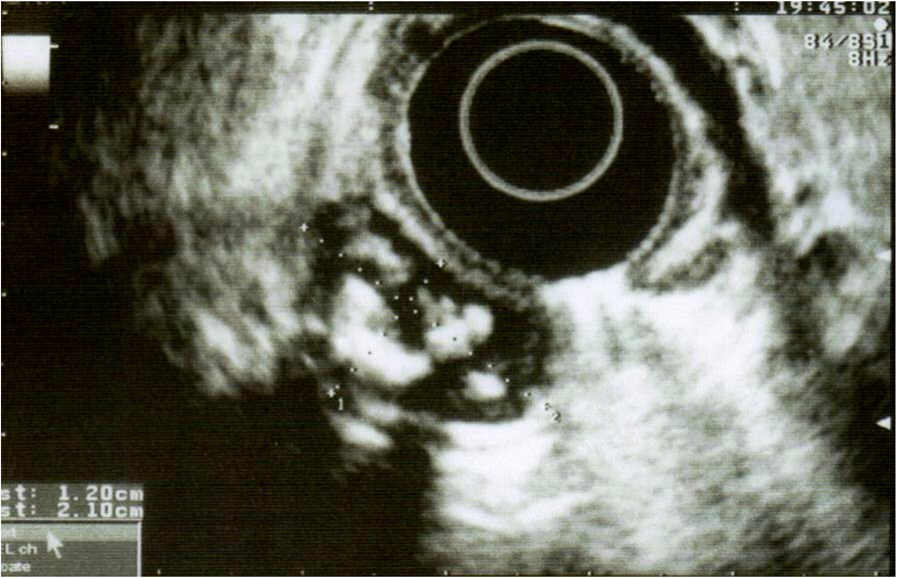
An endoscopic ultrasound showed a unilocular cystic lesion, developed in the muscularis, containing echogenic images.
